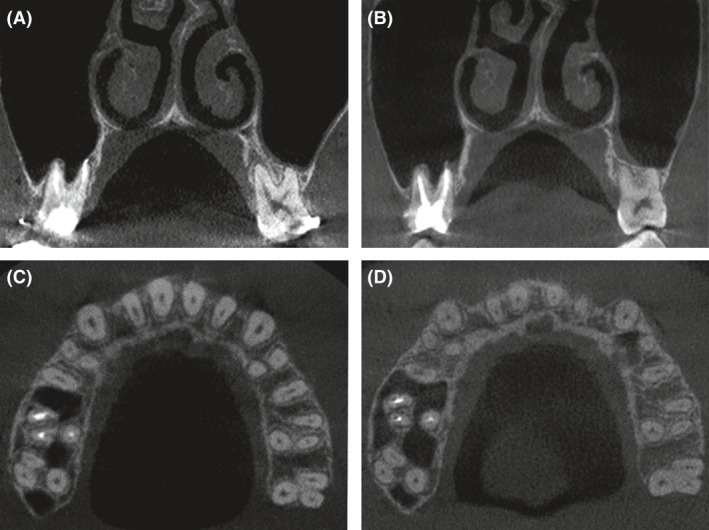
Cone‐beam computed tomography (CBCT) images. (A) CBCT image of the coronal section was taken before the RME to indicate the inclination of the maxillary first molars. (C) CBCT image of the axial section was taken before the RME at the one‐third part close to the root apex of the maxillary first molars. (B and D) The image of the coronal section was taken after the orthodontic treatment.
